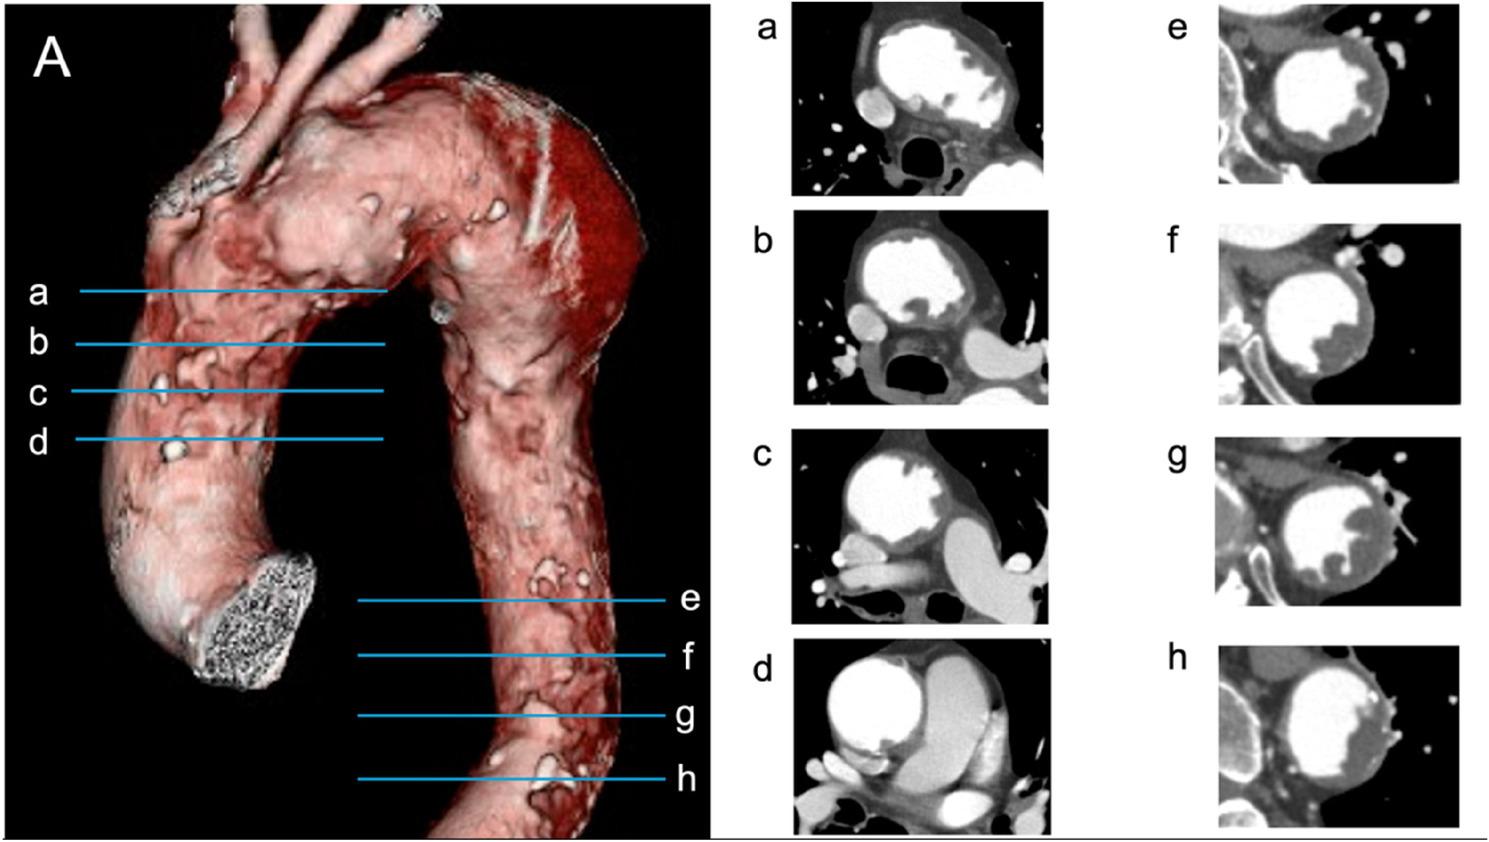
Contrast-enhanced computed tomography revealed an aortic arch aneurysm with extensive irregular intraluminal material, consistent with a shaggy aorta, extending from the ascending to the descending aorta
